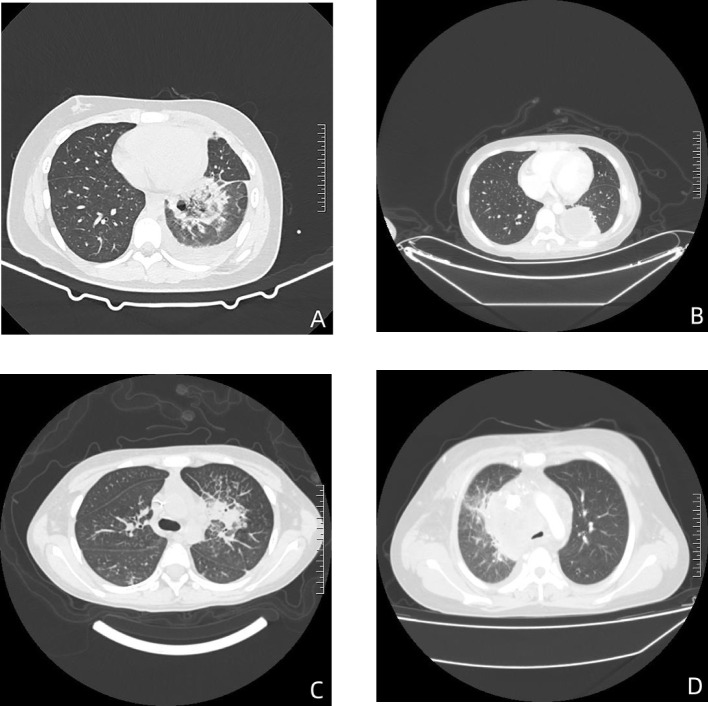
The chest CT findings of children with mucormycosis. (A) In the lower lobe of the left lung, there’s a mass-like area of high density with an internal cavity. This suggests a localized infection with tissue destruction leading to cavity formation. (B) In the lower left lung, there’s an encapsulated fluid density shadow accompanied by some consolidation. This could indicate an abscess or a localized collection of fluid, possibly due to infection. (C) There’s evidence of obstructive pneumonia in the upper lobe of the left lung, with partial narrowing of the upper lobe bronchus and multiple patchy areas of high density observed distally. This is indicative of infection causing obstruction in the airways. (D) In the mediastinum, there’s an unclearly demarcated mass-like soft tissue density shadow. Additionally, there’s narrowing and truncation of the right upper lobe bronchus and multiple solid nodules in both lungs. This could represent the spread of infection or inflammatory response in the mediastinum and lungs.
